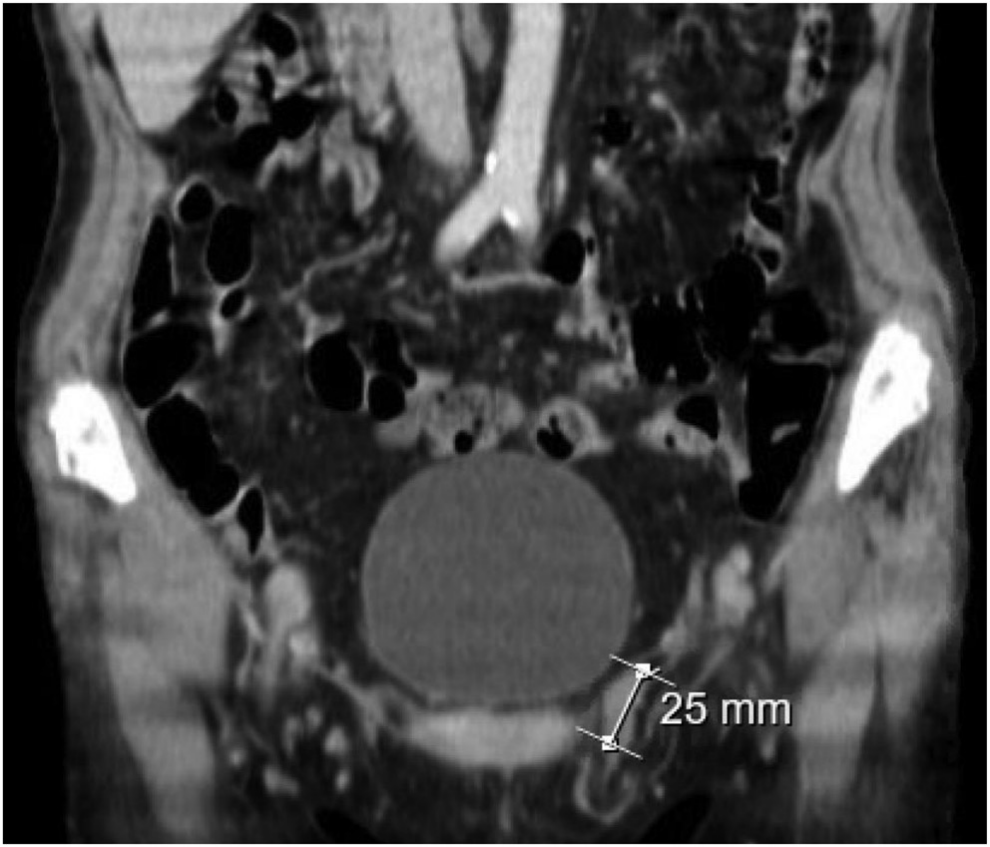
Abdominal CT scan showing a nodular lesion observed in the left inguinal canal with 25 × 10 mm, compatible with a testicular remnant.
